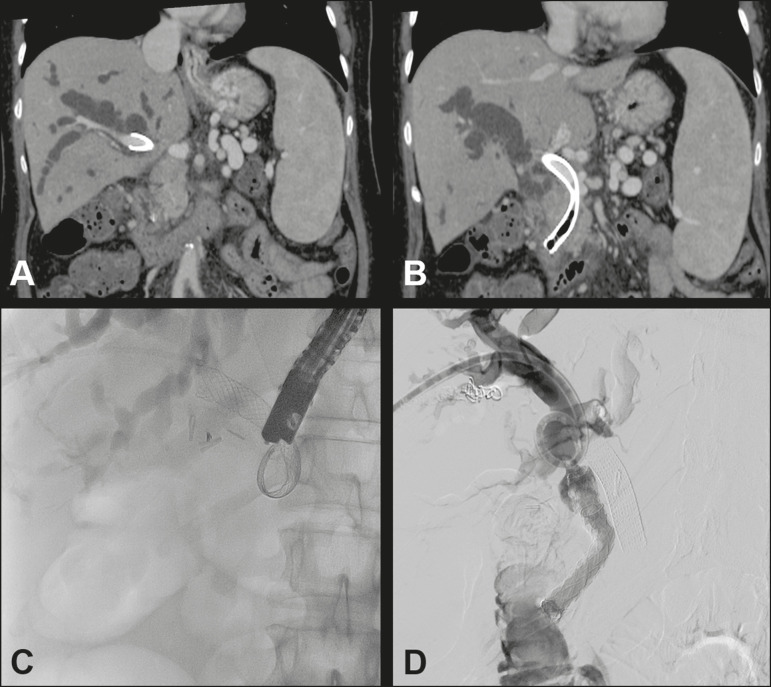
Female patient with locally advanced, inoperable pancreatic cancer. She underwent ERCP for the placement of a metal biliary stent, thereafter evolving to controlled hemorrhagic shock, followed by sepsis. Contrast-enhanced computed tomography of the abdomen and pelvis showed the proximal portion of the stent in the portal vein and its distal portion in the duodenum (A,B). We simultaneously performed procedures for percutaneous drainage of biliary ducts, removal of the porto-duodenal stent by ERCP, and percutaneous portal angioplasty for occlusion of the porto-duodenal fistula (C,D).
